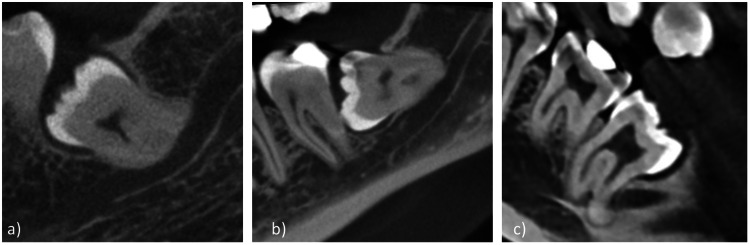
Images of various CBCT scans showing impacted third molars classified as (a) mesioangular, (b) horizontal, or (c) distoangular CBCT: cone-beam computed tomography
